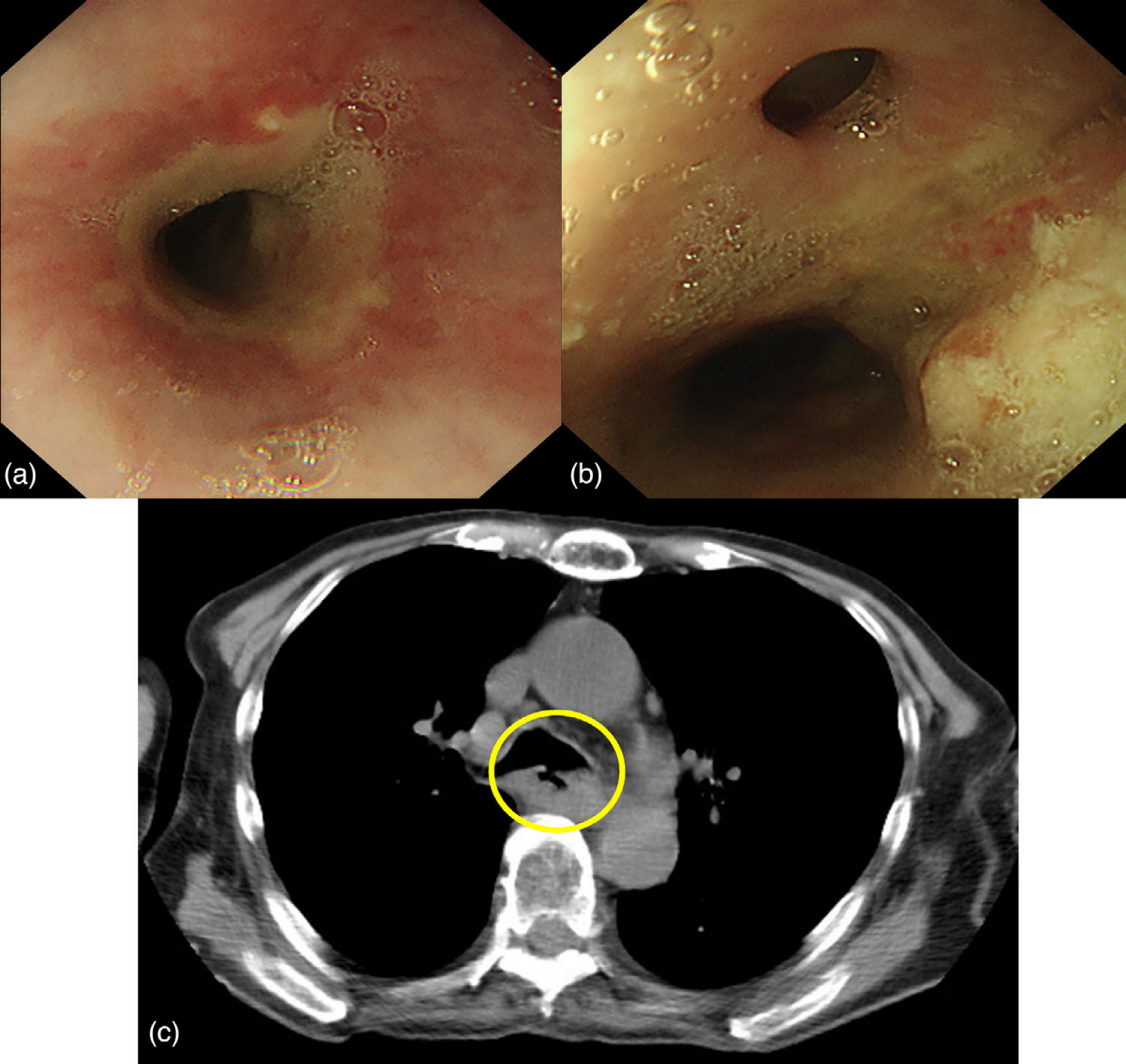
After radiotherapy for esophageal squamous cell carcinoma. (a) Upper gastrointestinal endoscopy shows a complete response to treatment for esophageal squamous cell carcinoma. (b) The fistula is confirmed in the middle esophagus. (c) Computed tomography shows esophageal and bronchial traffic (yellow circle).
